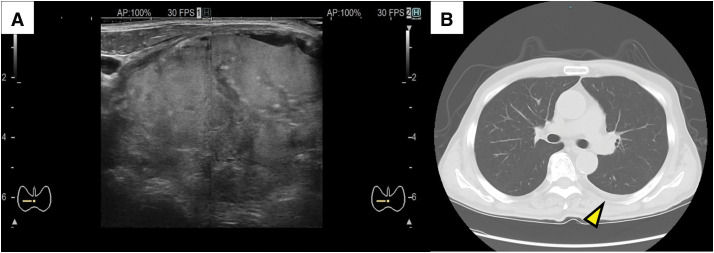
(A) Ultrasonography at the anterior neck showed a heterogeneous mass. (B) CT showed left pleural effusion (yellow arrowhead), but no pulmonary nodules.
